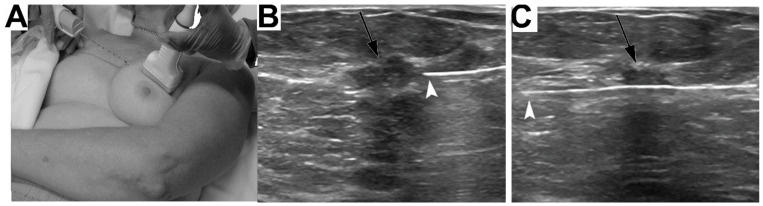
Image of ultrasound placement for ultrasound-guided biopsy. (A) Image of ultrasound-guided biopsy for medial breast lesions. (B) In the prefire position, needle tip (white arrowhead) should be placed at the margin of most lesions (black arrow), with needle parallel or near parallel to chest wall. (C) Postfire images should be documented and should definitively outline the needle traversing the lesion (black arrow). The tip of the needle may lie beyond the lesion margin in some cases, depending on the size of the target (white arrowhead).
